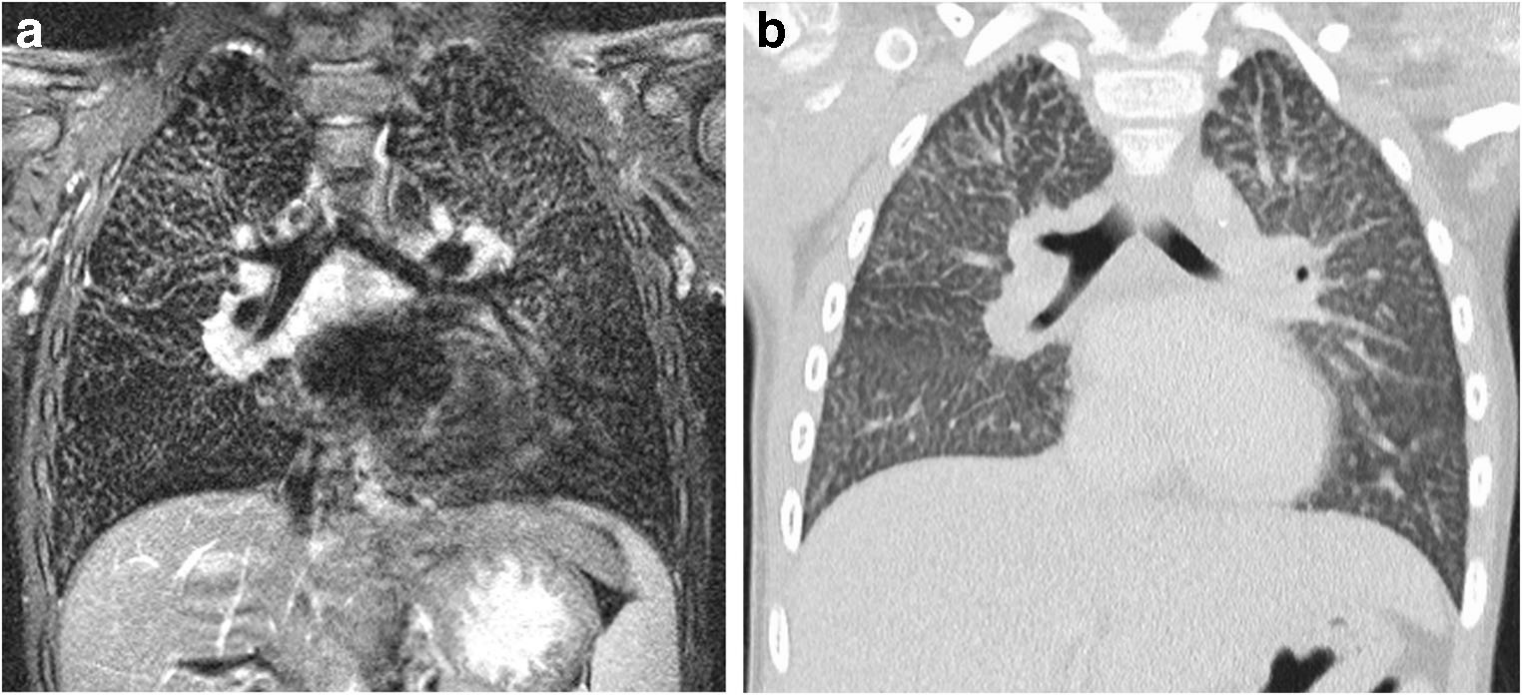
Interstitial lung disease in a 3-year-old boy. a Coronal T2-weighted turbo spin-echo MR image (parameters in Table 2) with 3-mm slice thickness at 3 T shows a diffuse ubiquitous fine granular pattern of interstitial lung disease. With a slice thickness of 5 mm, the fine granular interstitial process would hardly be recognizable if it affected the entire lung homogeneously. b Corresponding coronal CT image shows similar findings
